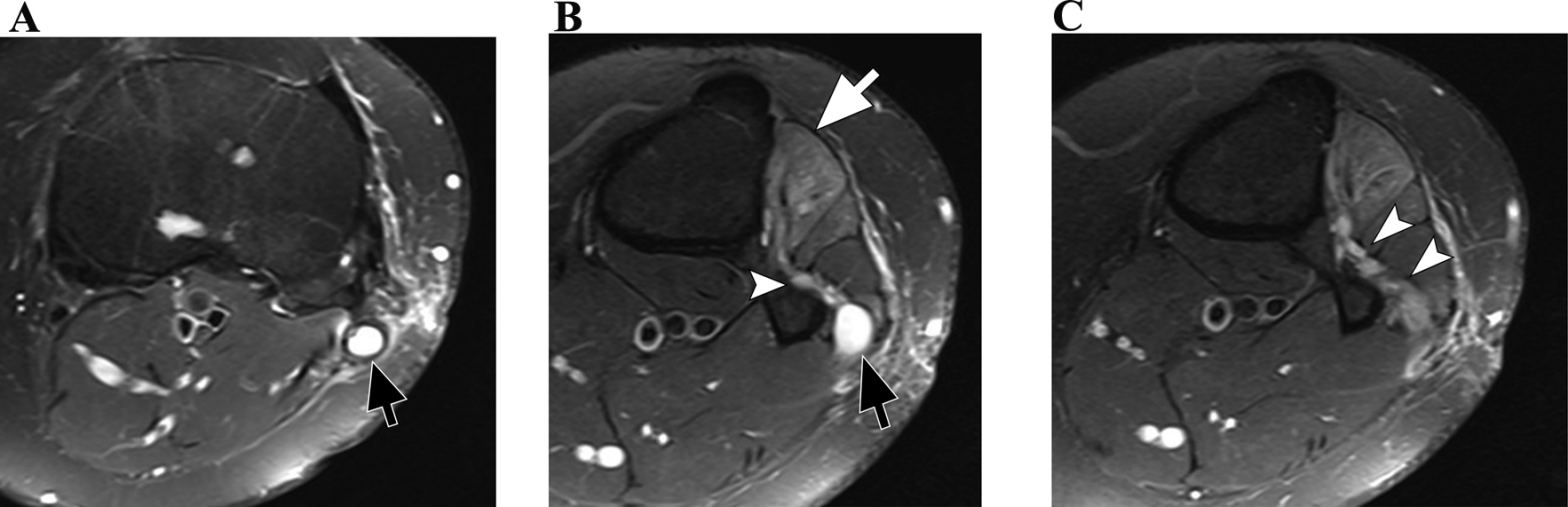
Axial T2-weighted consecutive images (A, B and C) of the left knee with fat suppression. There is a cyst within the common peroneal nerve (black arrows), originating from the proximal tibiofibular joint through its articular nerve branch (arrowheads). There is denervation oedema within the extensor muscles (white arrow)
